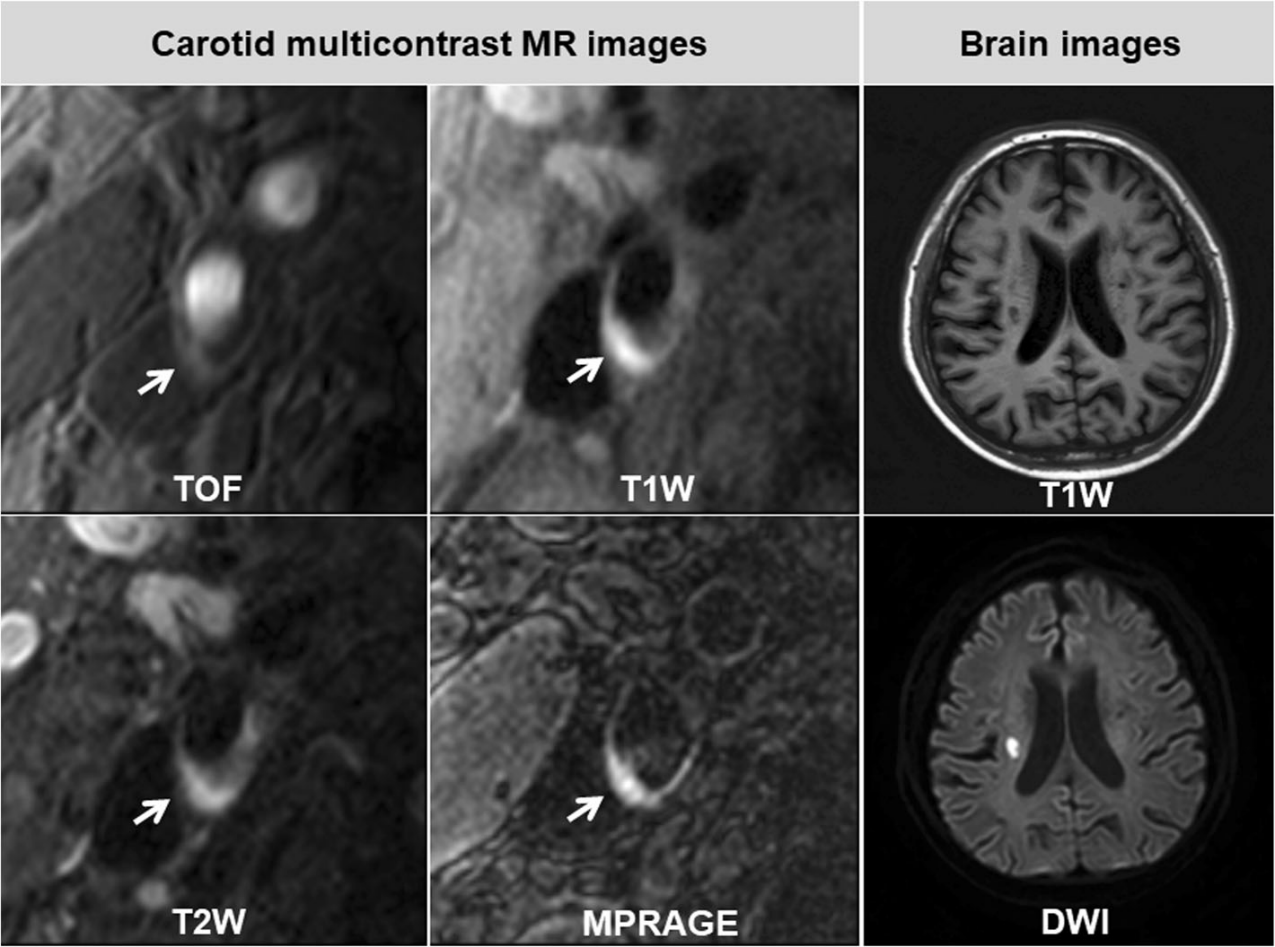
An example of a patient with carotid intraplaque hemorrhage (IPH) and ipsilateral acute cerebral infarct (ACI). Multicontrast MR images show an atherosclerotic plaque with large IPH (hyperintensity within the vessel wall, white arrows) in the right internal carotid artery. On Brain images of the same patient, acute cerebral infarct which shows hypointensity on T1W image and hyperintensity on DWI image can be seen in the right hemisphere
